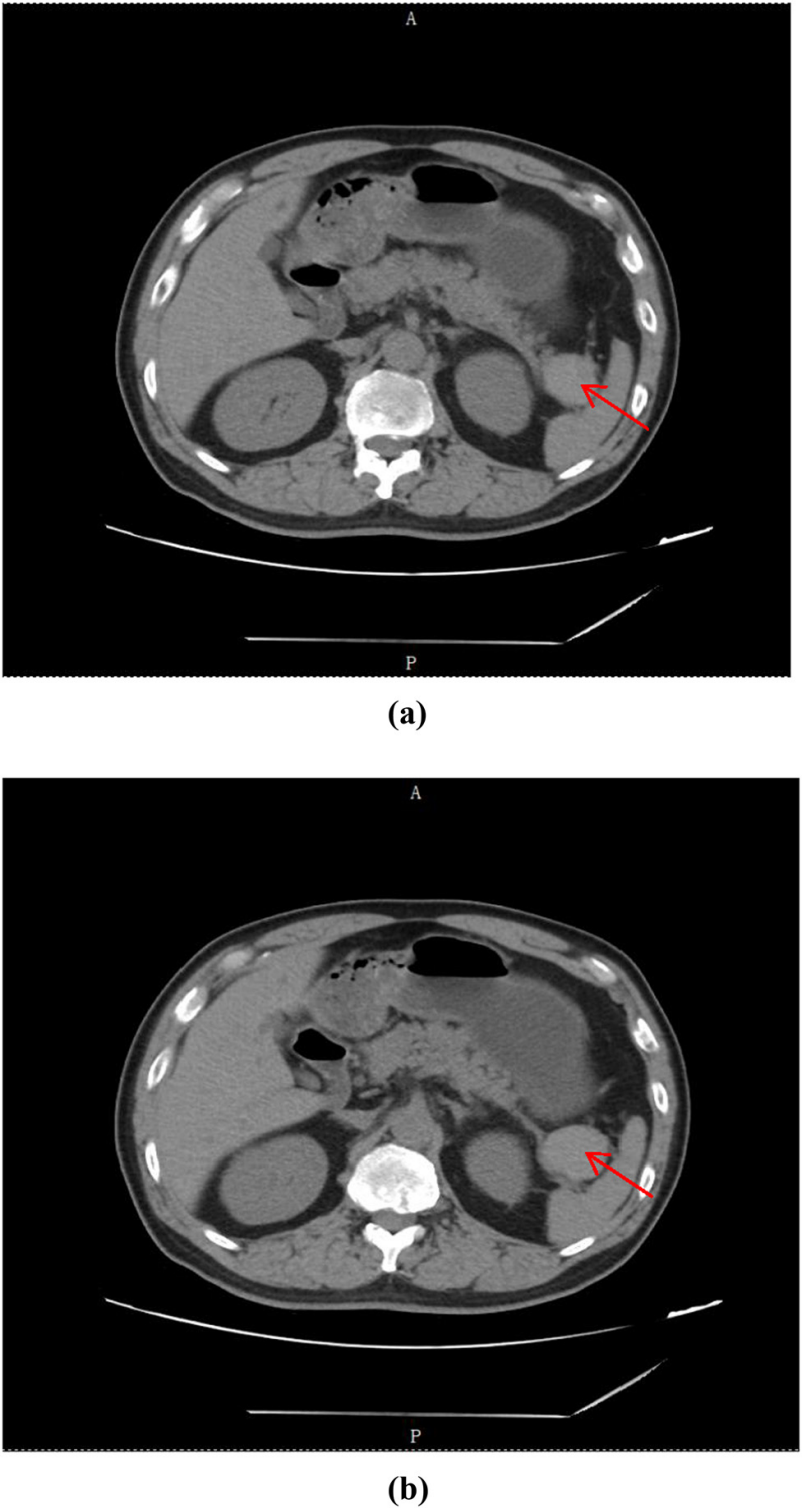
(a, b) CT scan of the abdominal space occupying lesion. The red arrow shows nearly-circular soft tissue density lesion as 88HU located at the spleen and stomach clearance.
